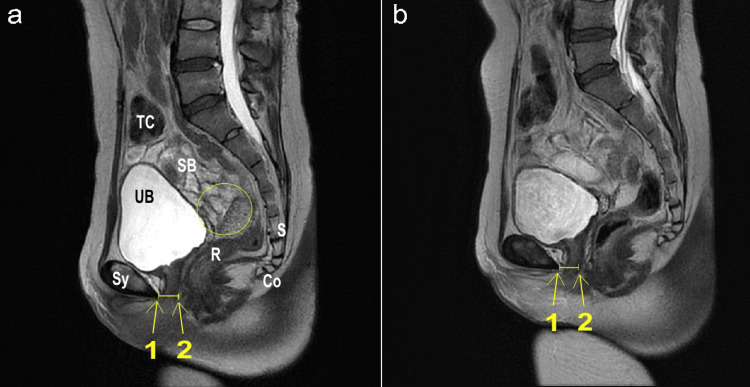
Changes in urethral thickness on T2-weighted sagittal view a: before treatment; b: after treatment Sy: symphysis pubis; UB: bladder; SB: small intestine; R: rectum; S: sacrum; Co: coccyx; TC: transverse colon Yellow open circle: normal location of the uterus The yellow line represents the distance between the posterior end of the pubic bone (1) and the posterior end of the urethra (2).
